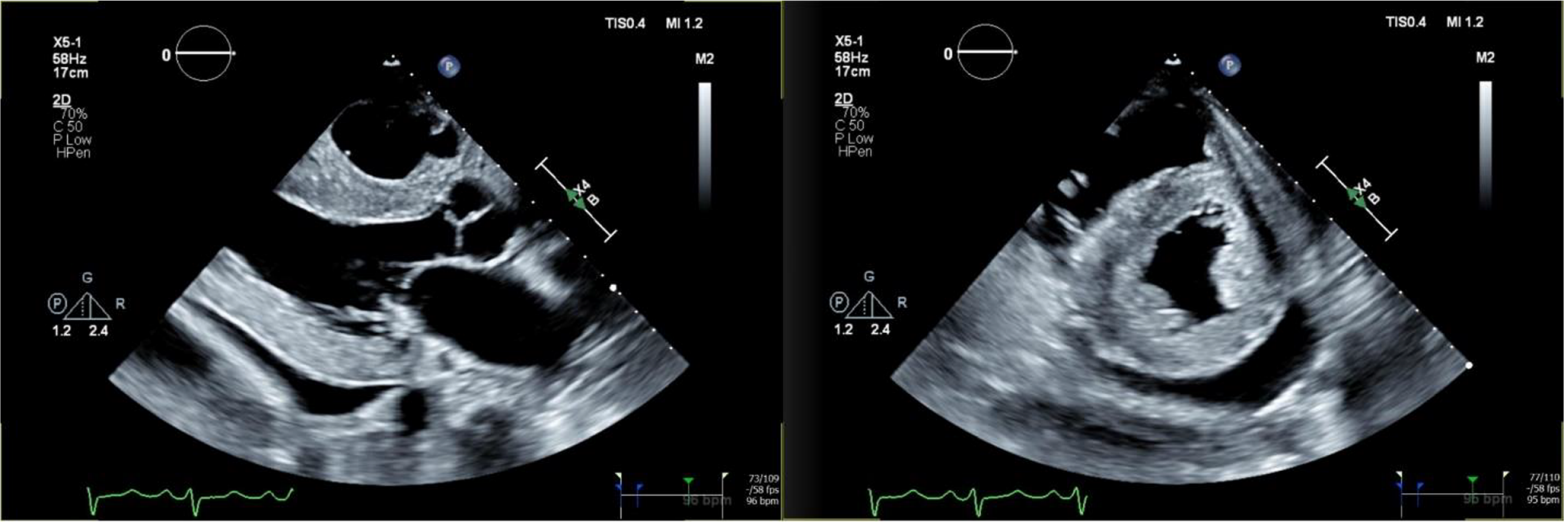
Parasternal long axis (left) and short axis (right) views of the heart demonstrate significant left ventricular hypertrophy with bright, sparkling left ventricular myocardium. A pericardial effusion is also evident posterior to the left ventricle
